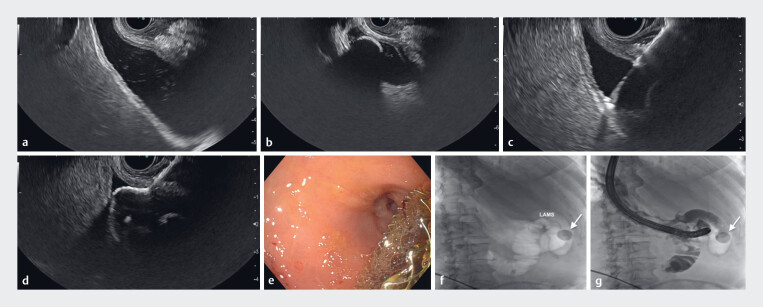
Images during endoscopic ultrasound (EUS)-guided cholecystoduodenostomy placement
